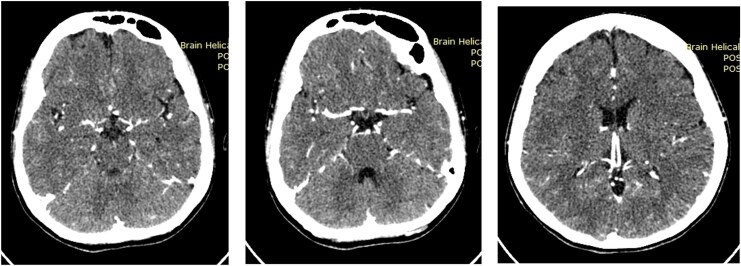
Cerebral CT angiography showed neither abnormalities nor cerebral aneurysms.
